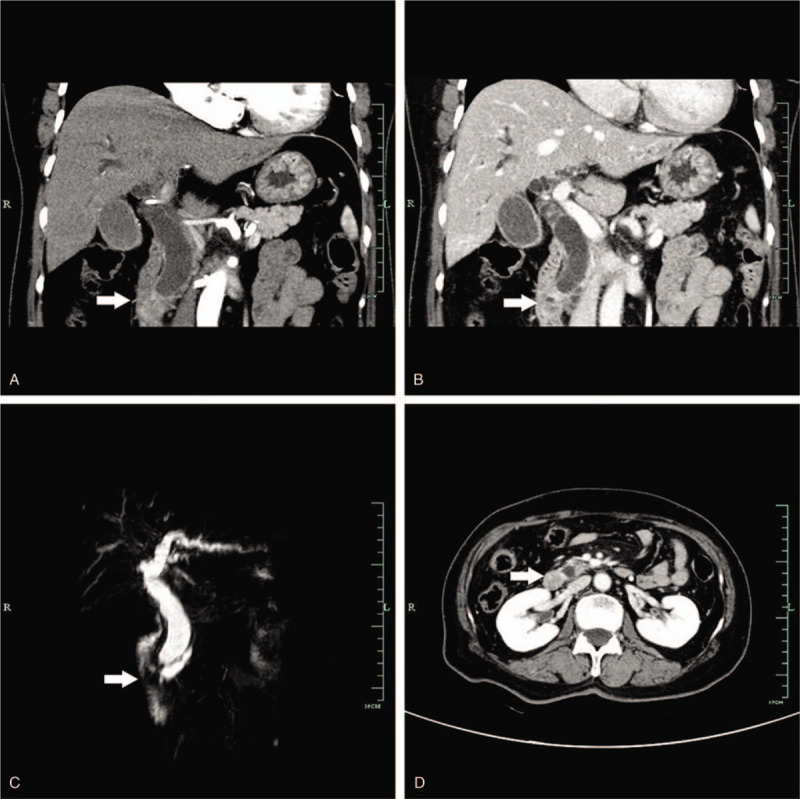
Enhanced CT of the abdomen and MRCP. Enhanced CT of the abdomen showed a dilated common bile duct and a moderately enhanced mass (arrow, 3 × 3 cm) in the area of the duodenal papilla (A-B: coronal view, D: horizontal view). MRCP identified a duodenal papilla mass with corresponding dilated common biliary tracts and main pancreatic ducts (C). CT = computed tomography, MRCP = magnetic resonance cholangiopancreatography.
